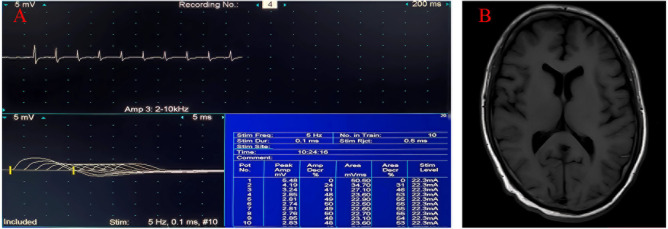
Clinical data of the patient 2. (A) Low-frequency stimulation of the left accessory nerve shows decreased waveform amplitude. (B) No abnormal signals are revealed by cranial magnetic resonance imaging.
